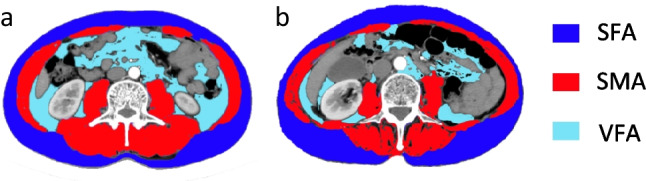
Measurement of the cross-sectional areas of skeletal muscle and fat at the L3 vertebral body level in CT scans of CRC patients with (a) and without (b) LNM. An axial CT image segmented into the visceral fat area (VFA), subcutaneous fat area (SFA), and skeletal muscle area (SMA)
